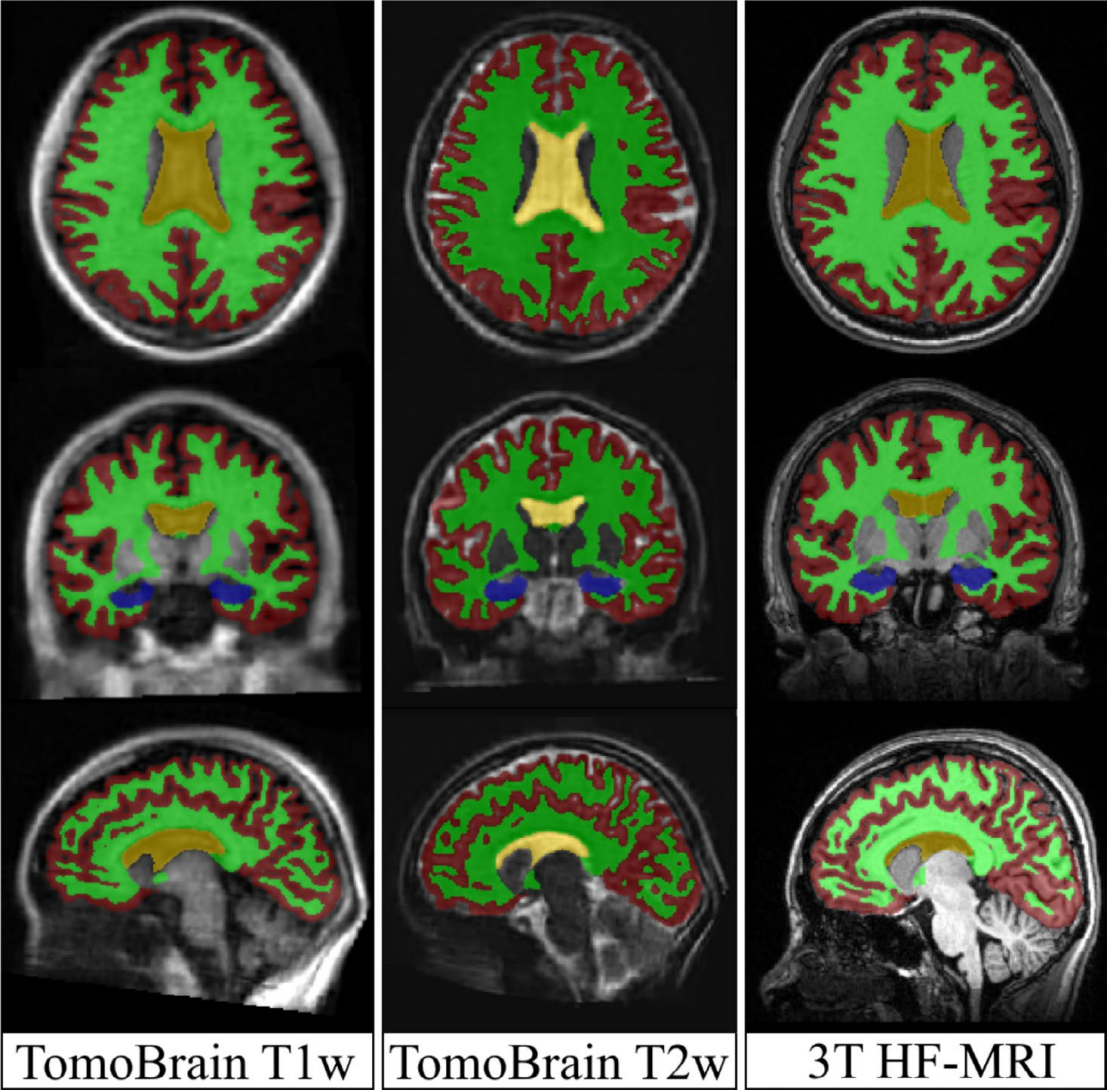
Example brain region segmentations made on the same subject on T1w *TomoBrain*, T2w *TomoBrain*, and 3 T HF‐MRI for gray matter (red), white matter (green), hippocampus (blue), and lateral ventricles (yellow).
